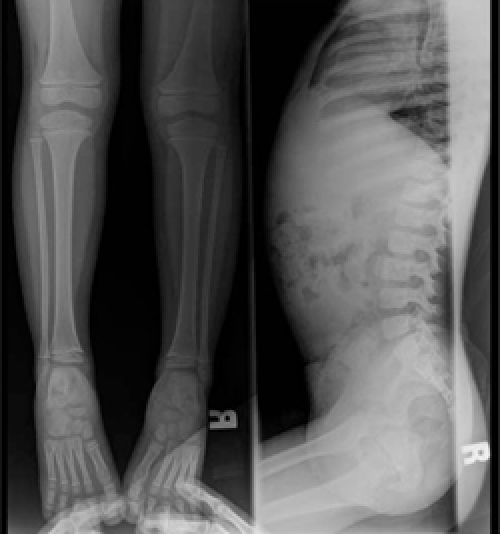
Figure 5. Radiography of the lower extremity and lateral vertebrae in Patient 2
